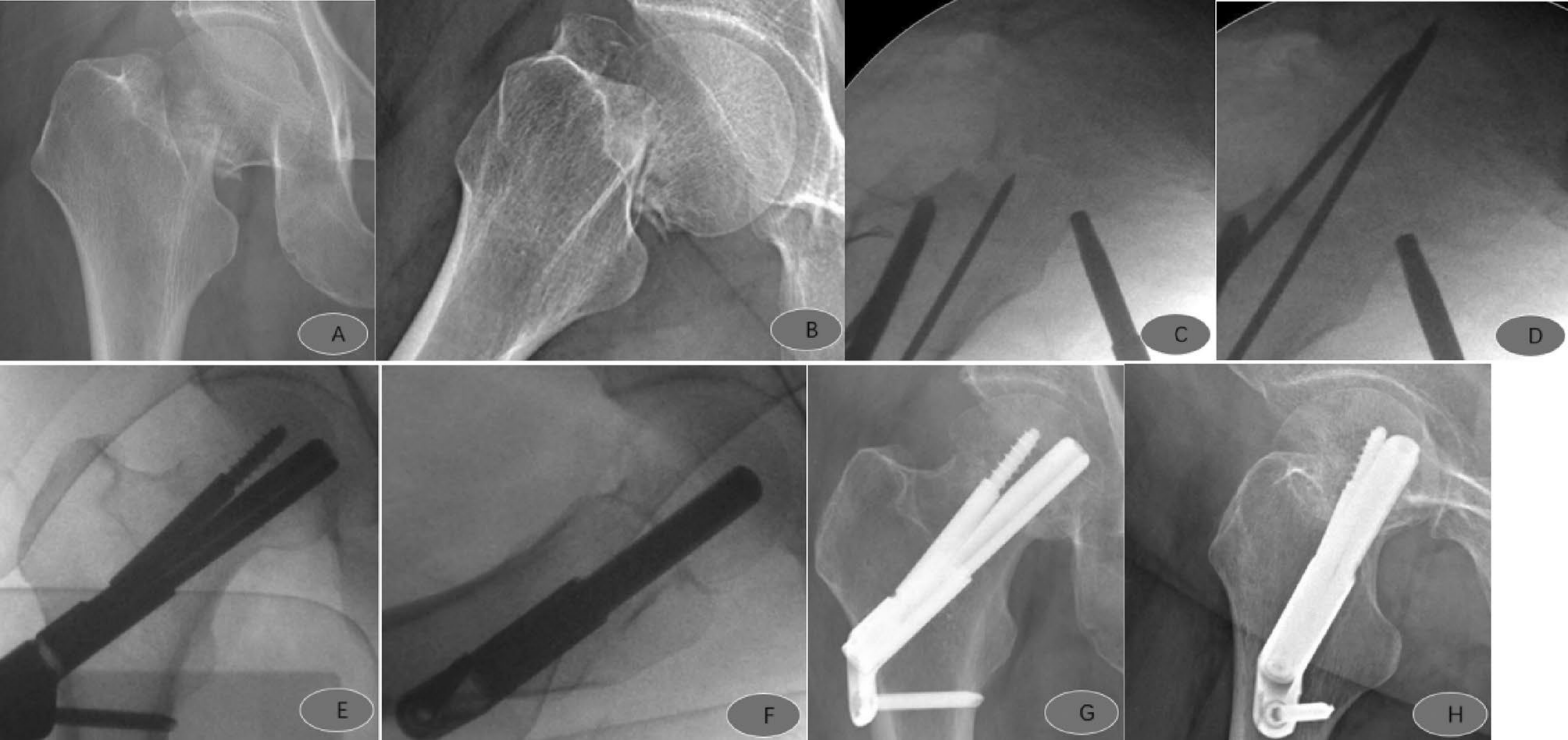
Female, 58 years old, admitted to the hospital with right hip pain and limited movement due to a fall; preoperative anteroposterior (A) and lateral (B) views showed a right femoral neck fracture, Garden type IV, type 3b; FNS was placed after satisfactory repositioning 5 days after the injury; intraoperative operations are shown in Figures (C, D, E, and F). Postoperative anteroposterior (F) and lateral (H) radiographs 3 months after surgery showed good fracture healing and satisfactory internal fixation position.
